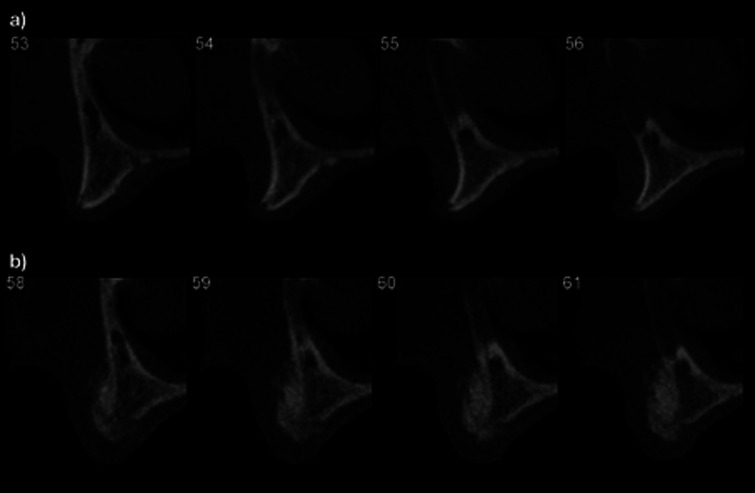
Tomographic images to be superposed for subsequent measurements. a) CBCT before bone graft, b) CBCT before implant placement.
